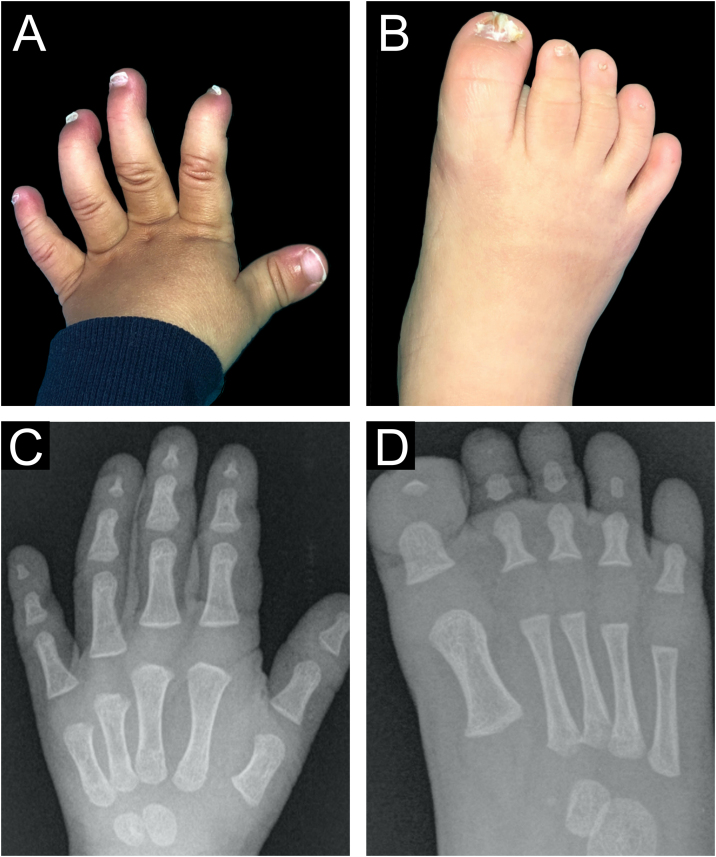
Clinical pictures with nail changes (A‒B) and radiographic (C‒D) images showing hypoplasia of the distal phalanges of the 2nd, 3rd, 4th, and 5th fingers, absent distal phalanges in the 2nd, 3rd, 4th, and 5th toes, and distal phalanx hypoplasia of the 1st toe.
